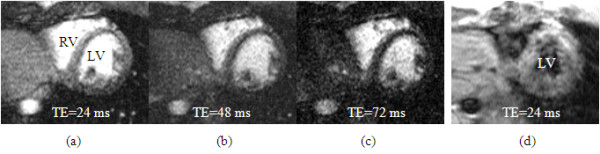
Examples of bright blood T2-weighted myocardial images at three different TEs, obtained from a volunteer during the adenosine infusion. In comparison, the TSE images collected is distorted by the fast heart rate and flow artifacts, which is not useful for further analysis.
